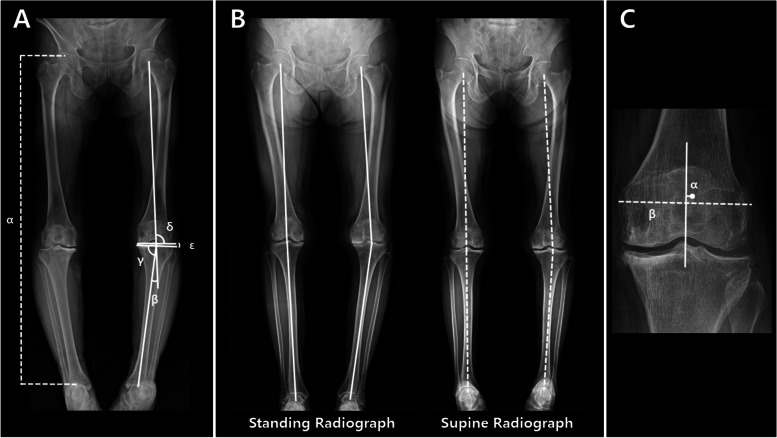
A Radiographic measurements of the length of the lower limb (α), HKA angle (β), medial proximal tibial angle (γ), lateral distal femoral angle (δ), and joint-line convergence angle (ε). B Full-length anteroposterior radiographs of the lower limb in both standing and supine position. The ΔHKA angle was defined as the absolute value of the difference between the acute angle generated by white solid lines and the acute angle formed by white dotted lines for each lower limb. C Patellar rotation was evaluated by calculating the degree of deviation of the patellar centre relative to the midpoint of the line connecting both femoral epicondyles (A/B * 100, %)
